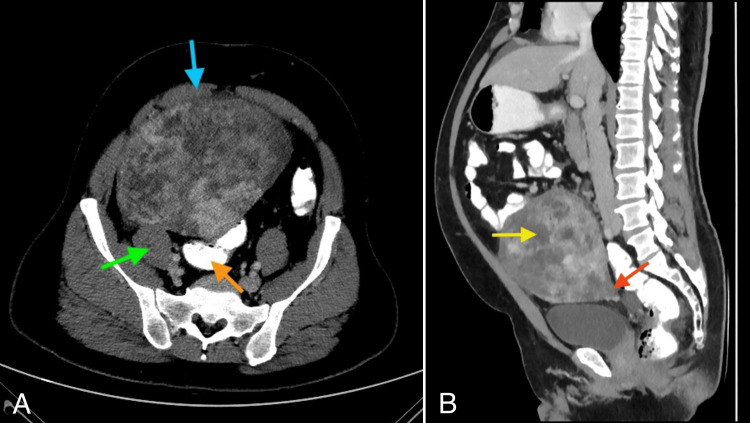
Computerized Tomography (CT) scan of the abdomen – axial section (A) and sagittal section (B). Axial section shows the tumor indenting the sigmoid colon posteriorly (orange arrow), psoas muscle posteriorly (green arrow), and abdomen wall anteriorly (blue arrow). Sagittal section shows the lower end of the mass (red arrow) supplied by branches from the inferior mesenteric artery through a pedicle near rectovesical region. A non-enhancing necrotic area (yellow arrow) is also noted.
